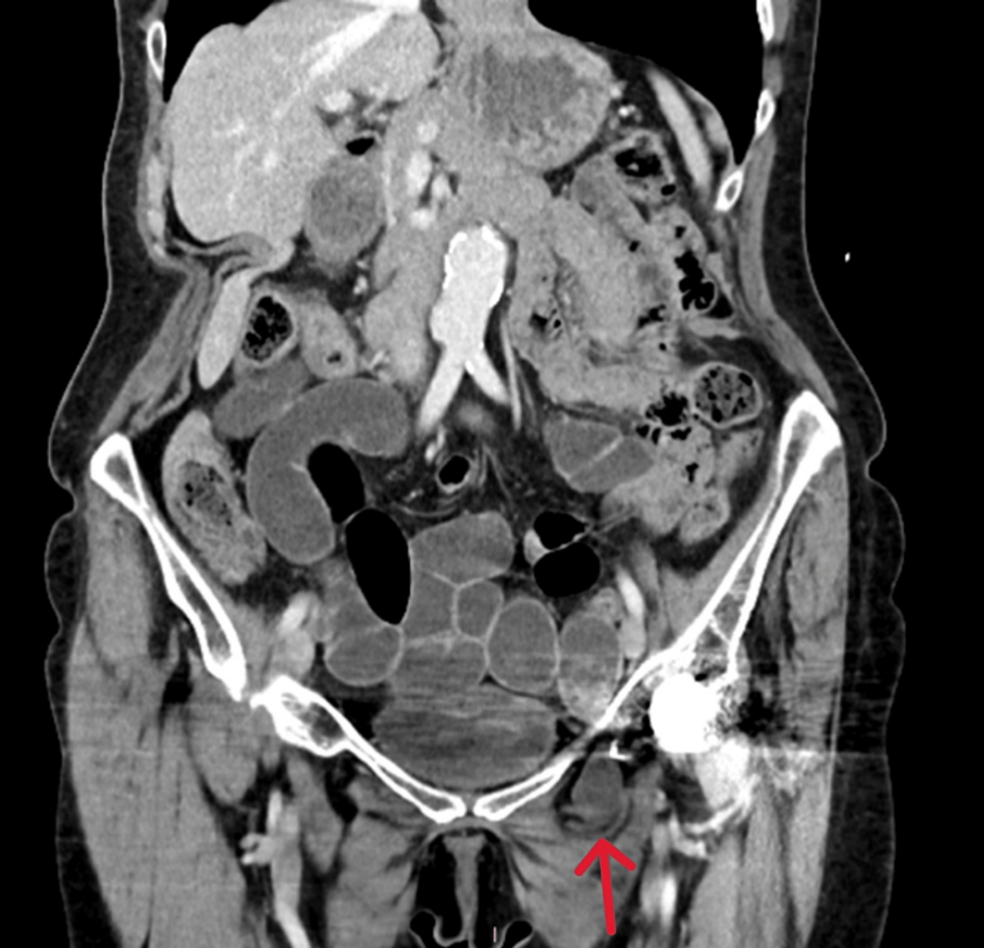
Abdominal coronal CT showing a left-sided obturator hernia (marked by arrow).
